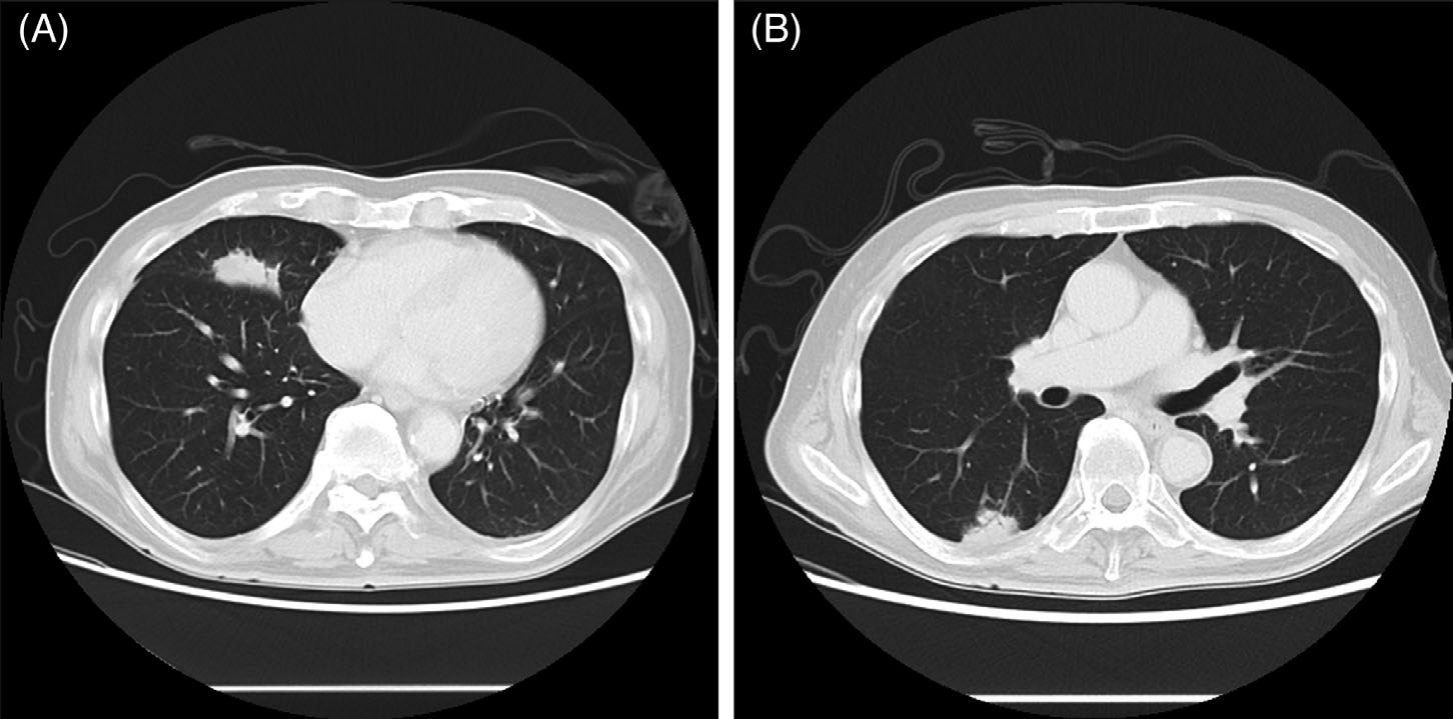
(A) Computed tomography (CT) of the chest shows an abnormal shadow in the right middle lobe 1 year after transcatheter arterial chemoembolization (TACE). (B) CT of the chest shows an abnormal shadow in the right lower lobe 3 years after TACE
